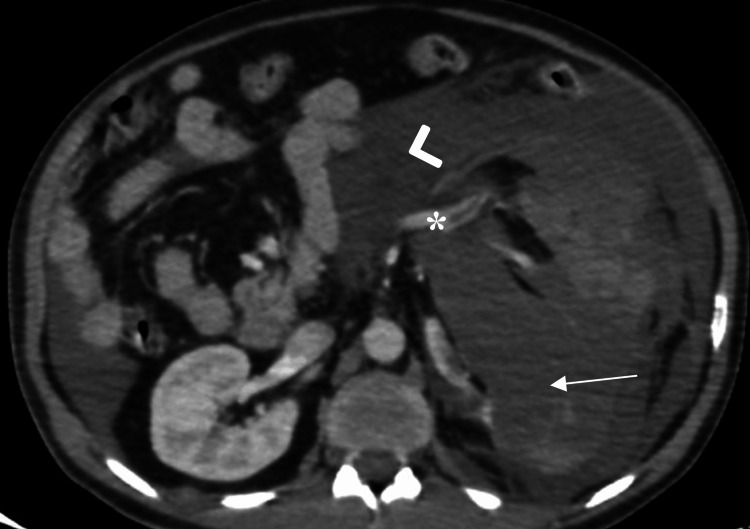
Axial contrast-enhanced computed tomography (CT) scan of the abdomen shows a shattered left kidney (arrow), with a large perinephric hematoma/urinoma (arrowhead), filling defect in the left renal vein indicative of vascular injury/thrombus (asterisk).
